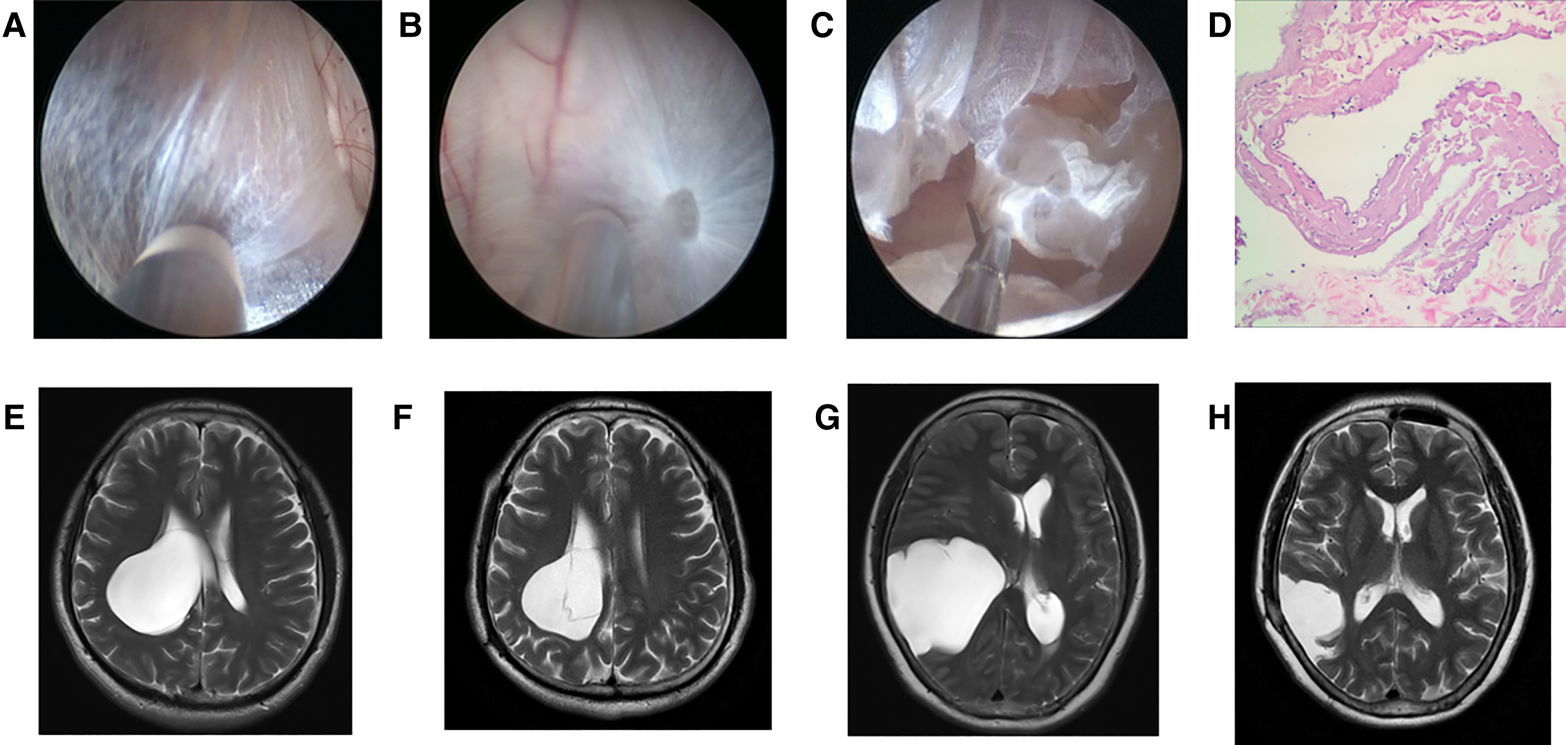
Neuroendoscopic treatment to intracranial arachnoid cyst. (A) Lateral wall of intracranial arachnoid cyst before it was incised (No. 6 patient). (B) Medial wall of intracranial arachnoid cyst after entering it with neuroendoscope (No. 6 patient). (C) Part of medial wall was resected with microshear (No. 6 patient). (D) Pathological image of the arachnoid cyst wall which was resected. (E) Preoperative T2 MRI image of No. 6 patient. (F) T2 MRI image of No. 6 patient at 2 years and 2 months after neuroendoscopic surgery. (G) Preoperative T2 MRI image of No. 5 patient. (H) T2 MRI image of No. 5 patient at 1 year after neuroendoscopic surgery.
